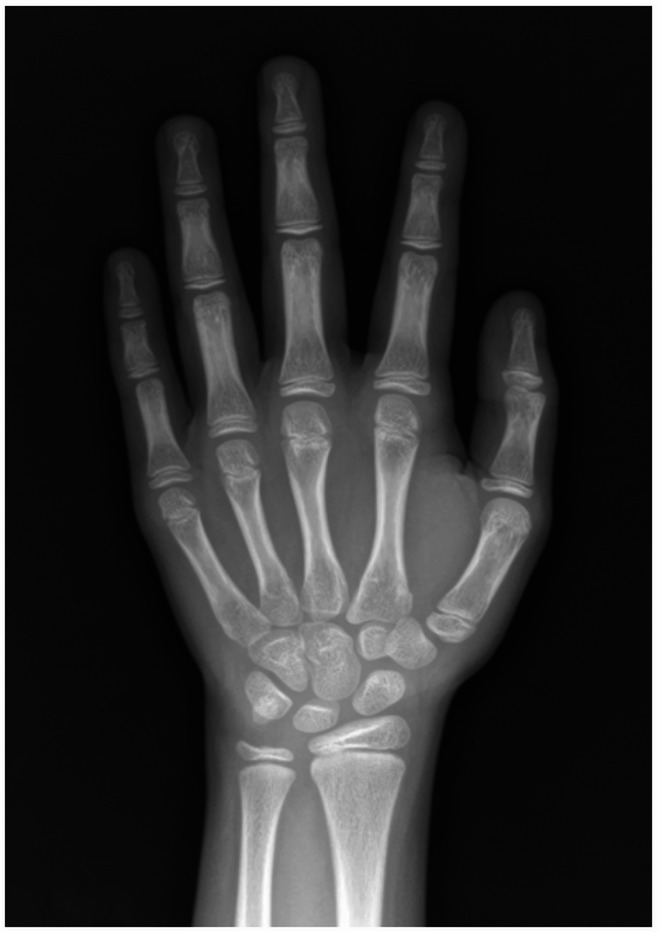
Inter-reader discrepancy in a hand radiograph of a female child. GP estimates among readers were 8 years 10 months, 11 years 0 months, and 10 years 0 months, whereas TW3-RUS estimates were 9 years 8 months, 10 years 0 months, and 10 years 0 months
